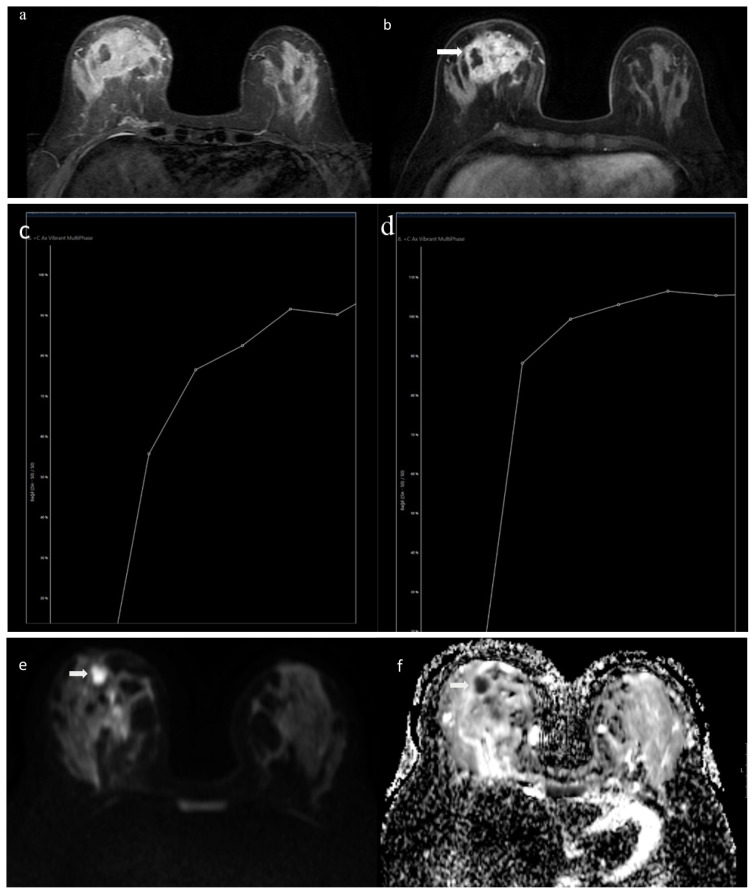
A 37-year-old female mother with right breast lump and bilateral breast pain. Axial STIR-weighted MR image shows a 5.5 × 4 cm (APxKK), hyperintense, mass-like lesion at right breast. 1b. Axial fat supressed T1-weighted MR image shows regional heterogeneous contrast enhancement including abscess formation (white arrow). 1c. Time intensity curve demonstrate progressive increase (Type I) contrast pattern. 1 d. Time intensity curve demonstrate plateau (Type II) contrast pattern. 1e–1f. Diffusion weighted MR images show the restriction of diffusion (white arrows).
